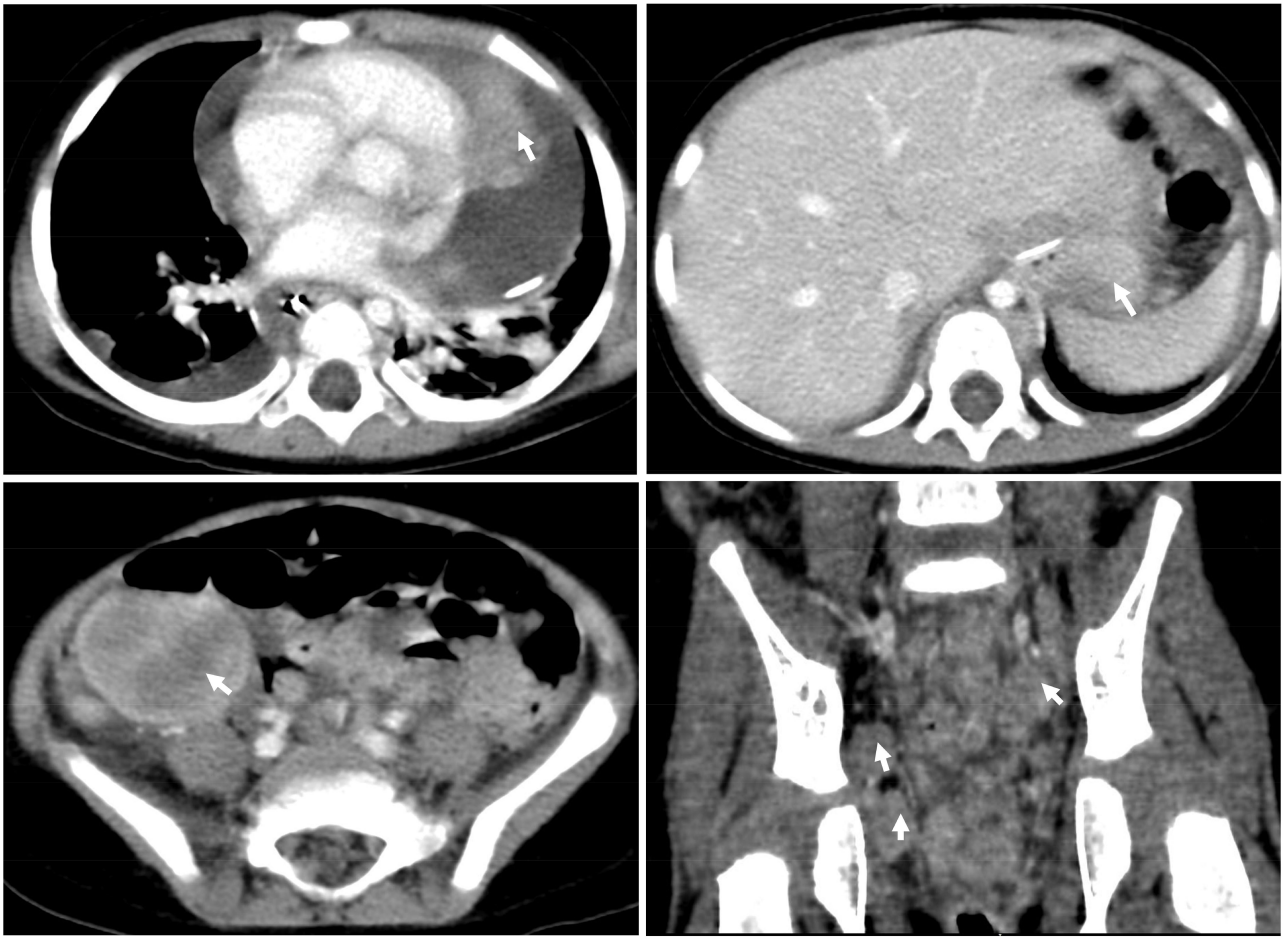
CT scan of the chest and abdomen at diagnosis. CT showed that not only the heart but also the chest, abdomen, and pelvis were involved.
